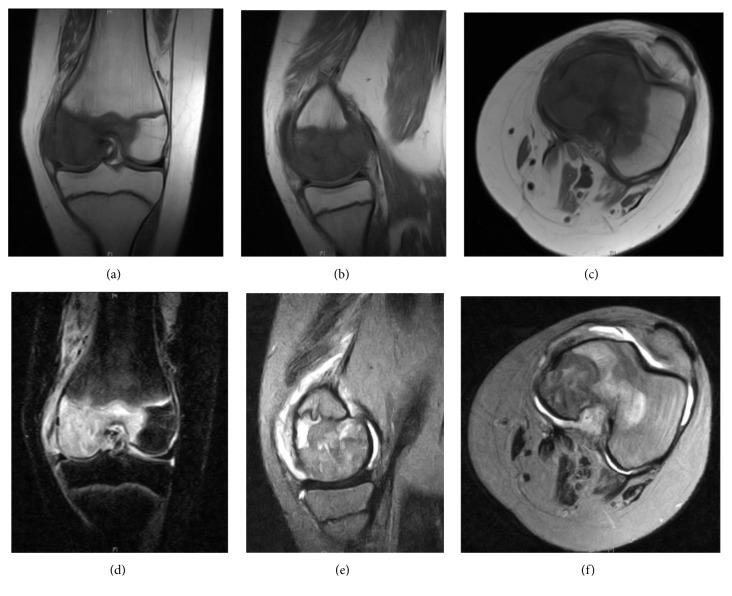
Magnetic resonance images (MRI): T1 coronal, sagittal, and axial views (a, b, c), STIR coronal (d), T2 sagittal (e), and axial views (f) of the left knee, July 2009.
